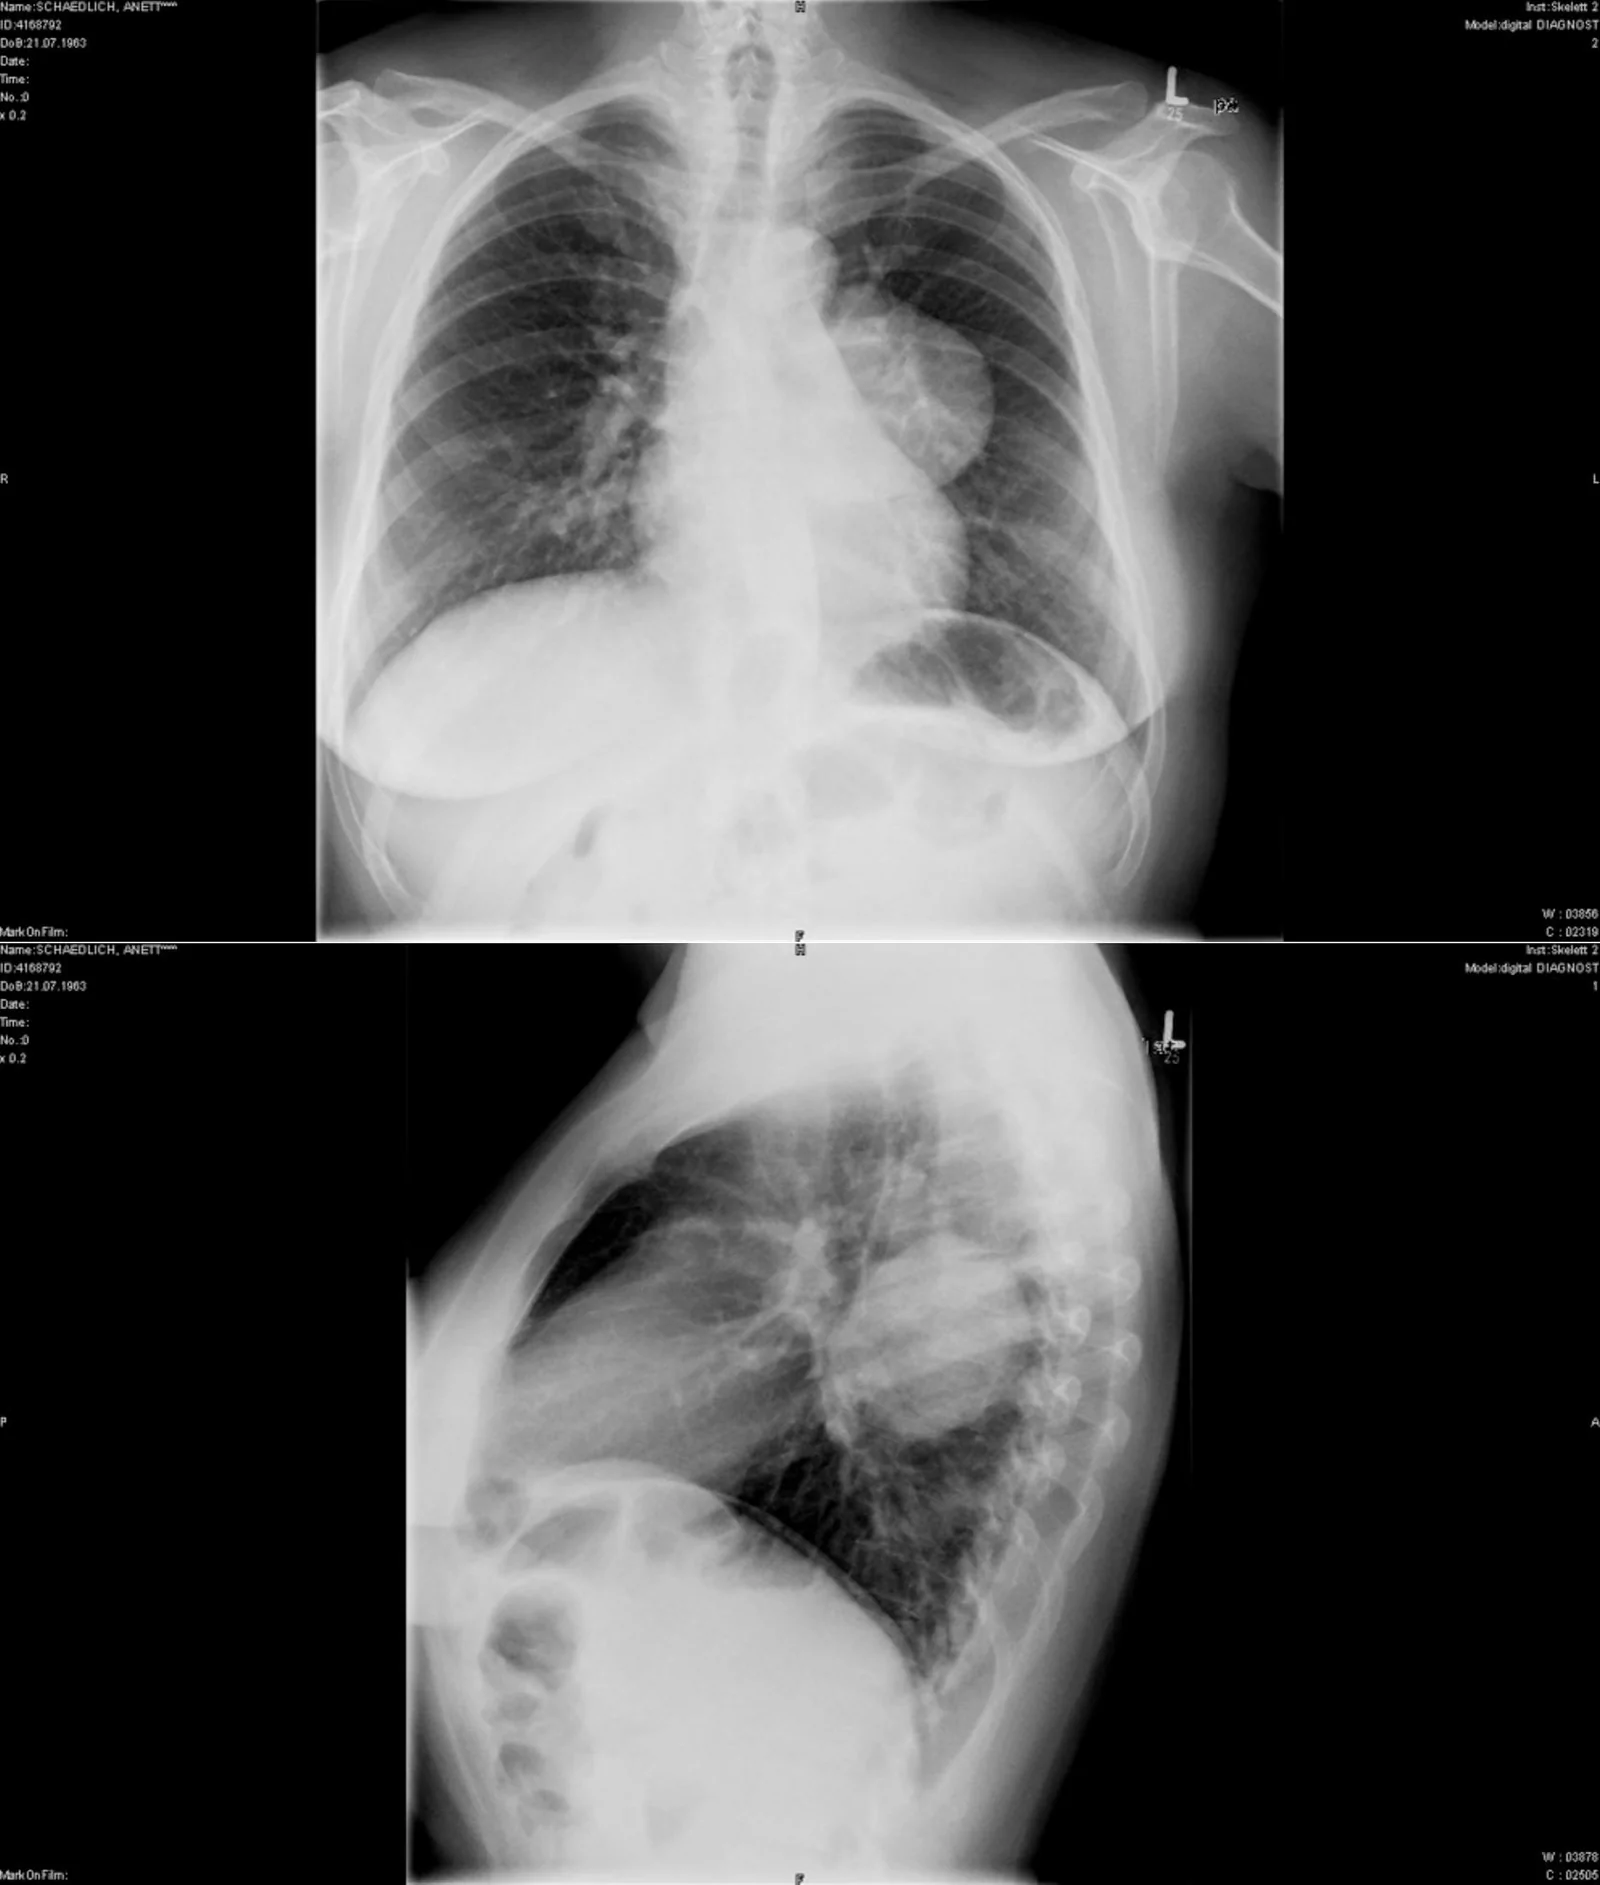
Chest x-ray showing left thoracic mass.
